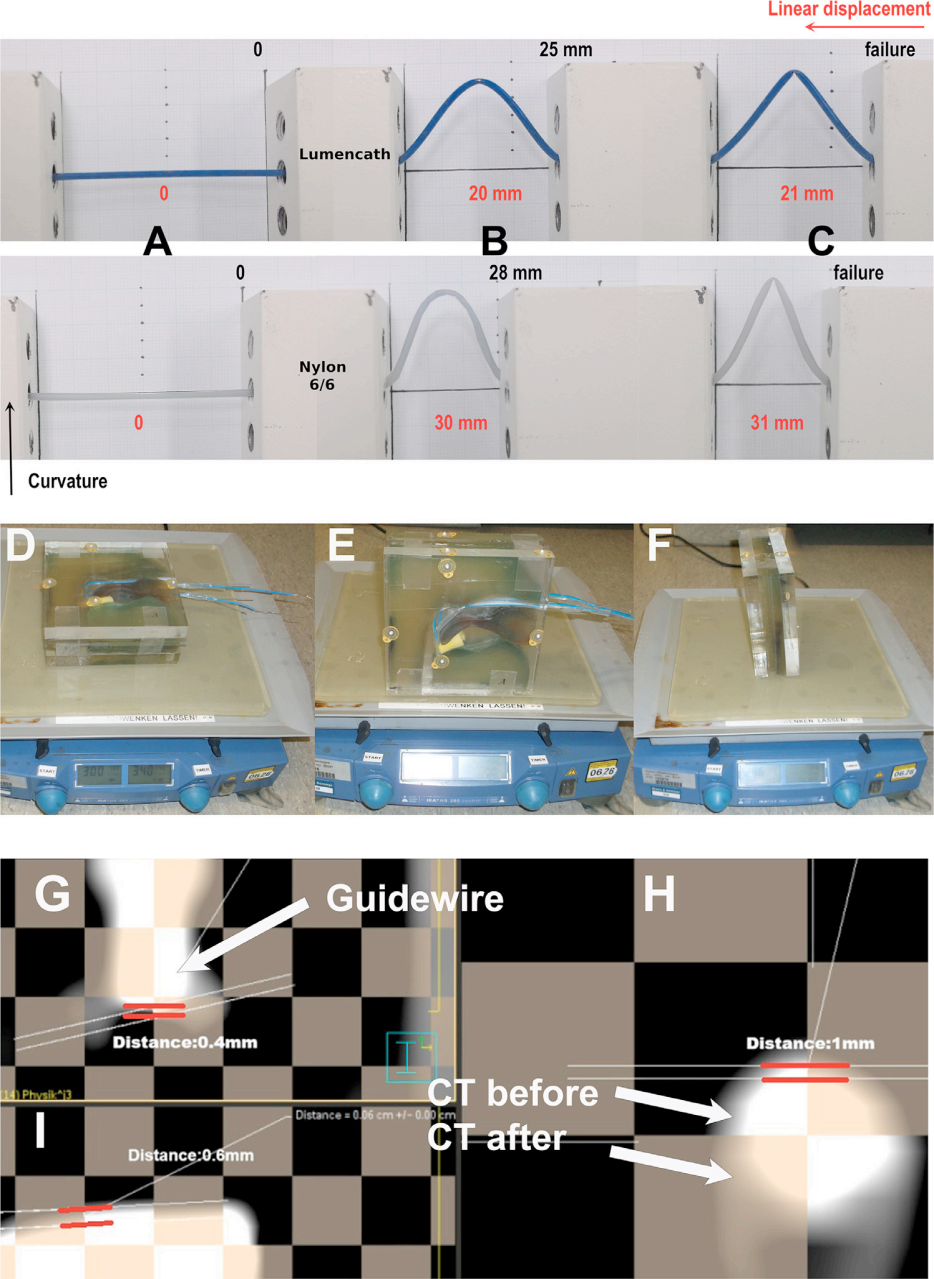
Mechanical and vibration testing of ANA with CT comparisons. *Abbreviations:* ANA = Augsburg Nasopharyngeal Applicator; CT = computed tomography.
